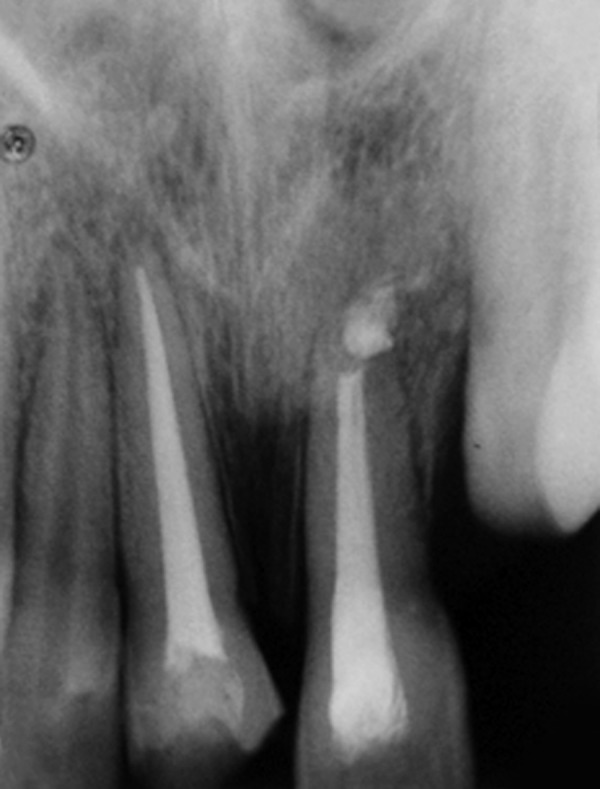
Postoperative intraoral periapical radiograph (case 3)
